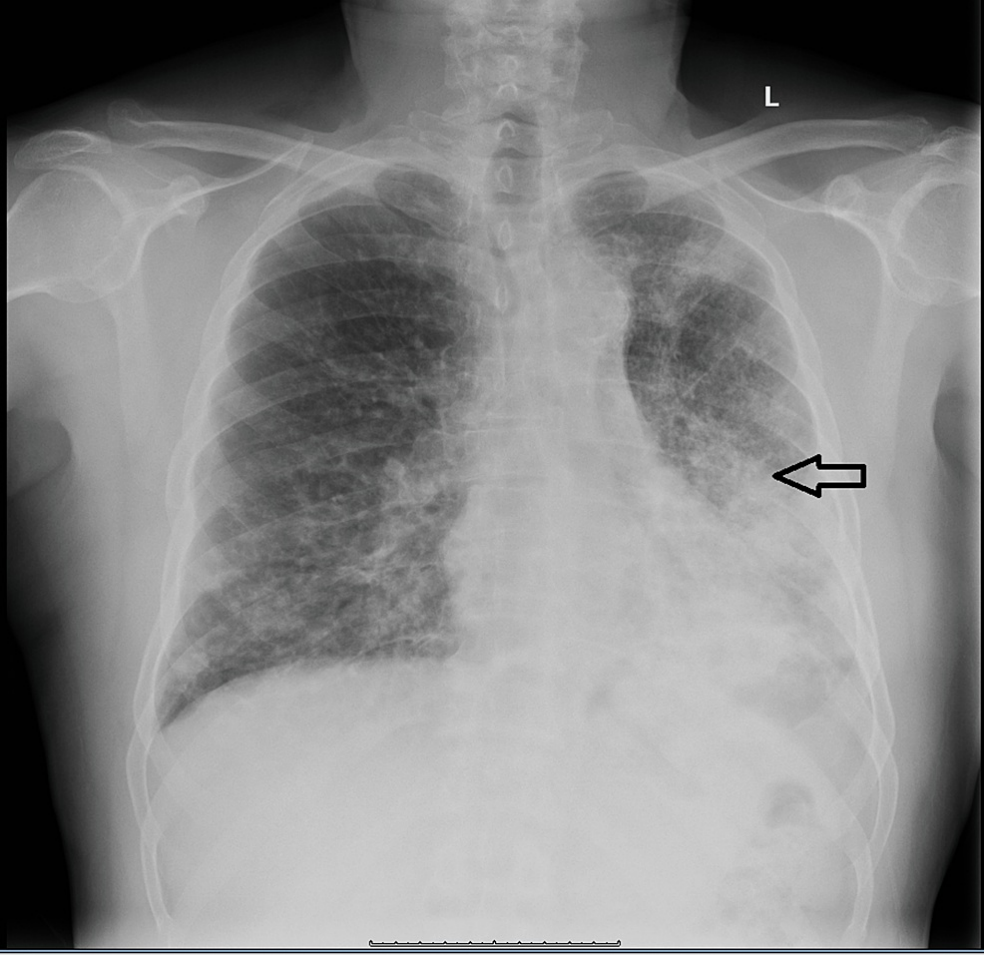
Chest x-ray posteroanterior view shows extensive patchy-to-confluent left-sided infiltrates. These are most severe in the left mid to lower lung fields with also significant patchy density in the left upper lobe. There are reticulonodular opacities involving right lower lung zone.
